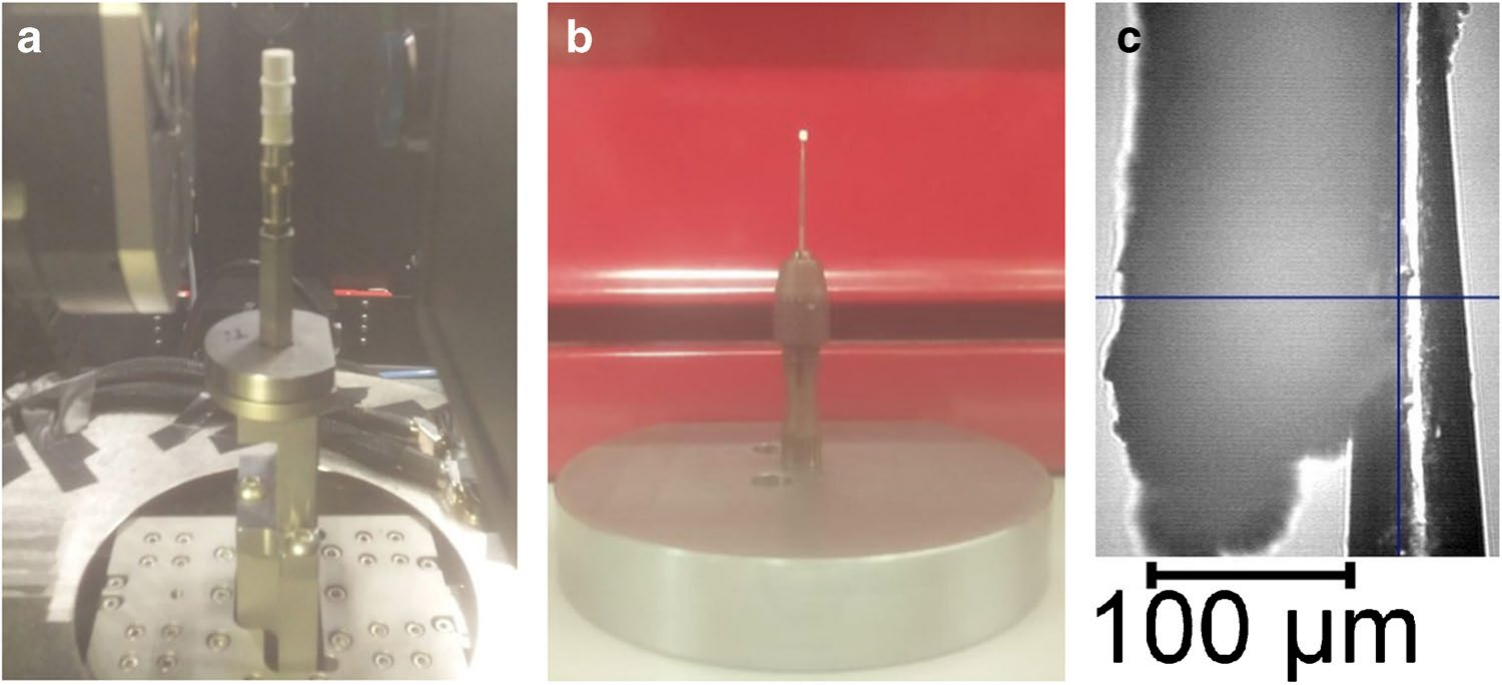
X-ray CT imaging setup. a Three mounted gyroids before whole-body scanning. b 3D-printed material cut-out adhered to the top of a pinhead and sample holder. c Magnified image of the interface between sample (left) and sample-holding pinhead (right)
